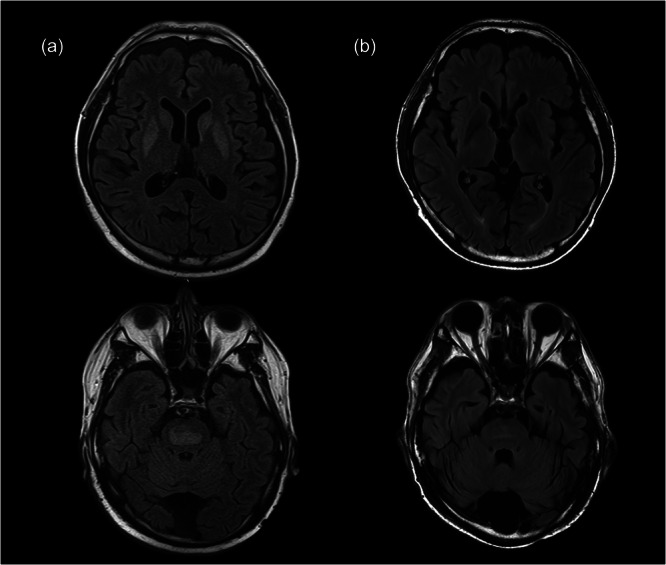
FLAIR images of this patient. (a) was performed in 2015 and (b) was performed in 2024. Hyperintensity in the basal ganglia and the central pons showed improvement.
